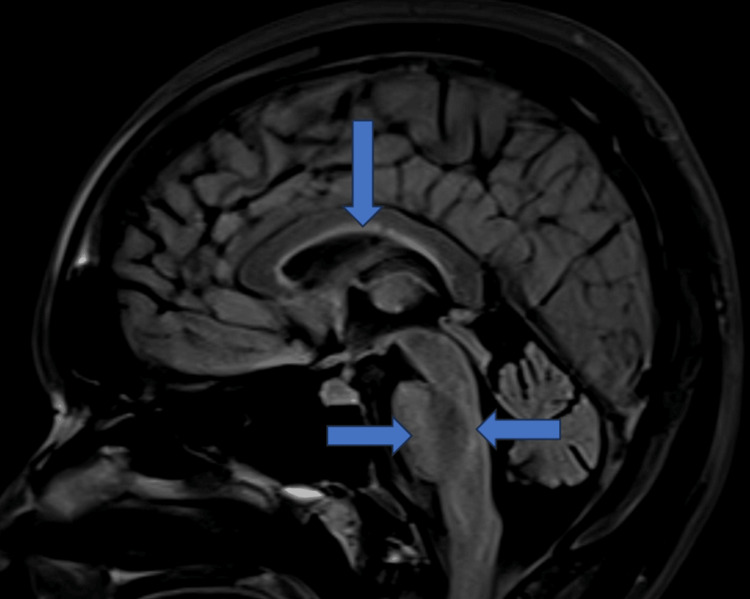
MRI (sagittal) showing T2 FLAIR hyperintensities in the corpus callosum, and periventricular white matter in the pons and medulla (red arrows) T2-FLAIR, T2-weighted fluid-attenuated inversion recovery
